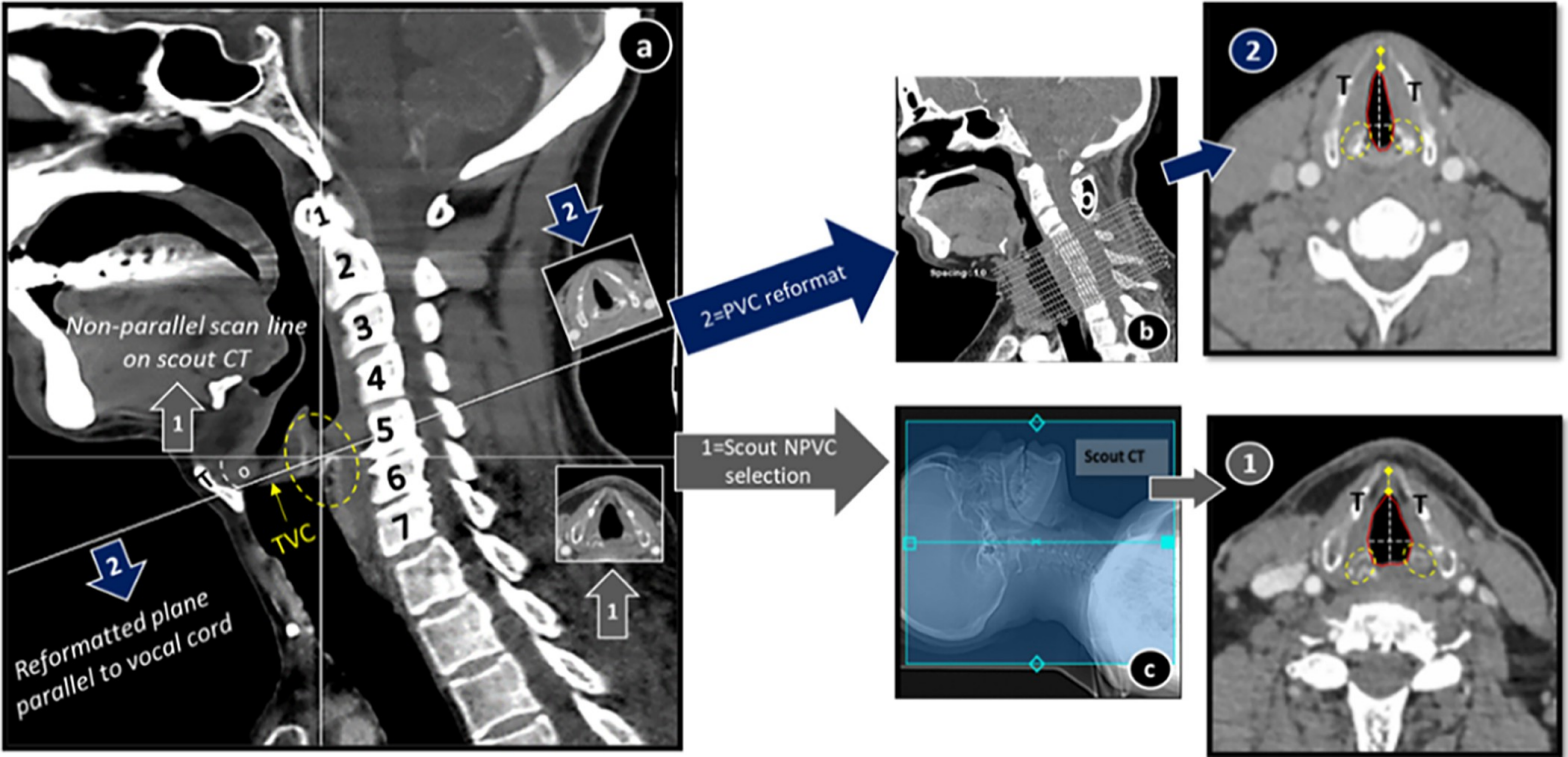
CT scan neck sagittal section (a) showing how glottic anatomy was studied in two axial planes, non-parallel, NPVC ①and parallel to vocal cord PVC ②. Scout CT (c) is showing predefined non-parallel CT scan lines and reformatted CT scan (b) showing PVC reformation lines in sagittal. Qualitative and quantitative parameters of glottis noted in both ① and ②. Dotted oval = cricoarytenoid joint (a,1,2), o = angulation between two planes (a), TVC = true vocal cord, red lumen trace = Cross-sectional area, dotted white lines = anteroposterior and transverse dimensions, double arrow line = anterior commisure, yellow dots = region of vocal cord width measurement.
